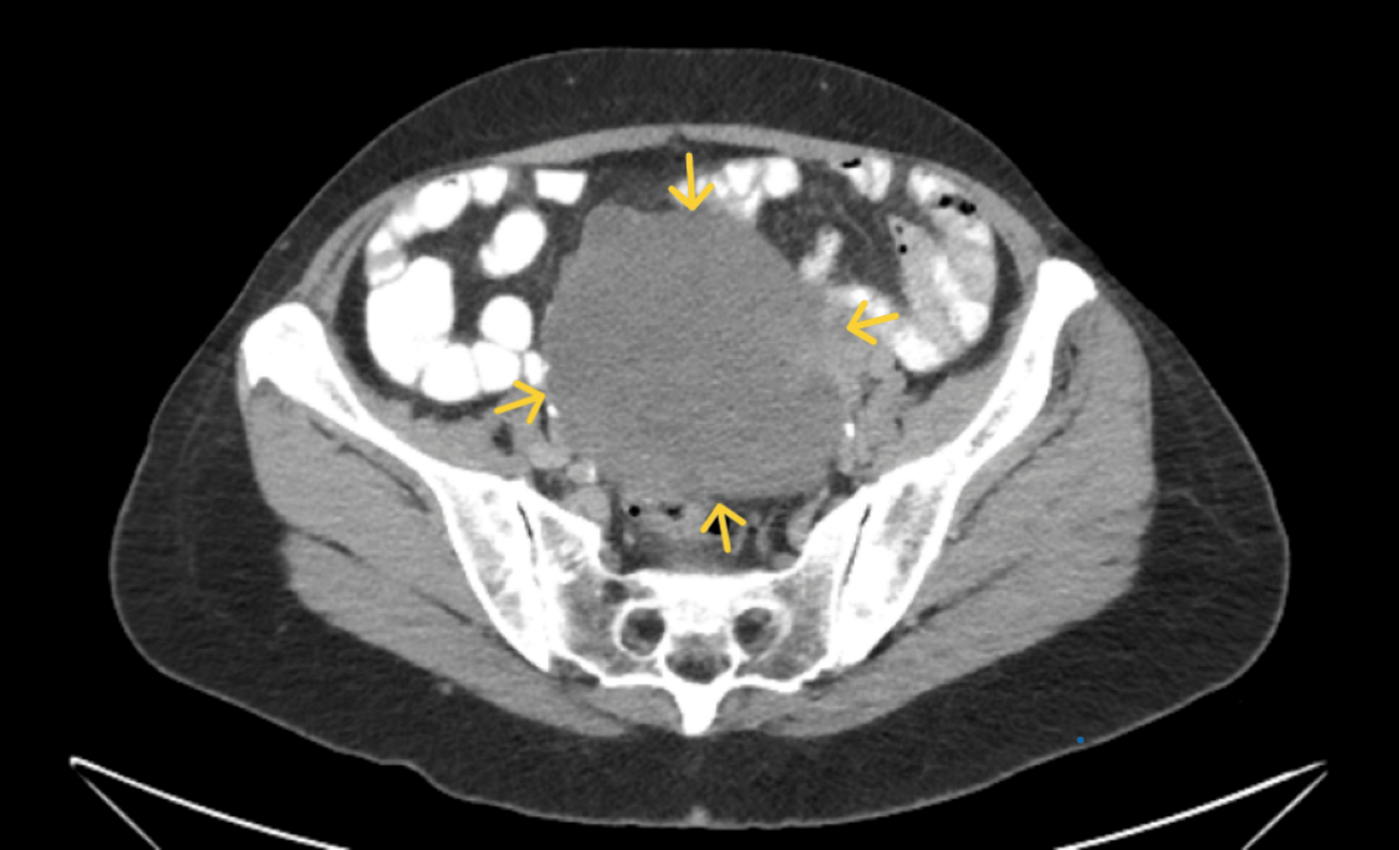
CT imaging of the ovarian thecoma A large mass with heterogeneous enhancement is evident (yellow arrows), originating from the left ovary and occupying the Douglas space
